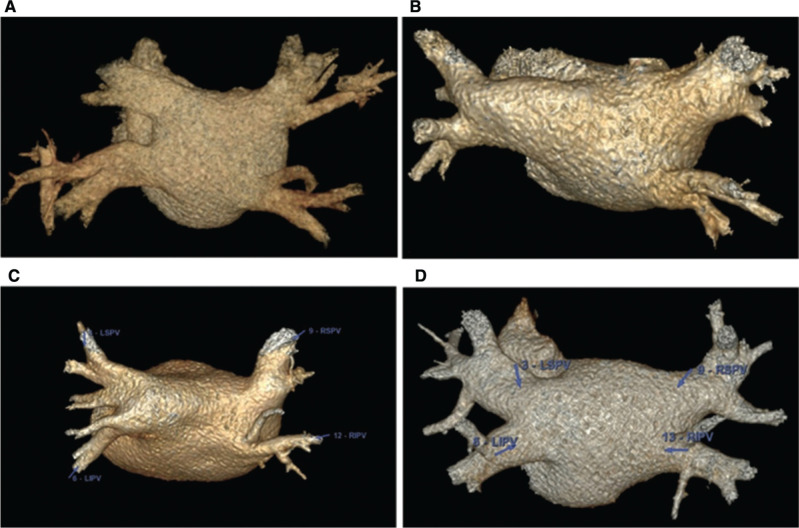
Cardiac computed tomography demonstrating variations in pulmonary venous anatomy. A: Two left pulmonary veins and 2 right pulmonary veins. B: One common left pulmonary vein and 2 right pulmonary veins. C: Two left pulmonary veins with early branching and 2 right pulmonary veins. D: Two left pulmonary veins and 3 right pulmonary veins.
